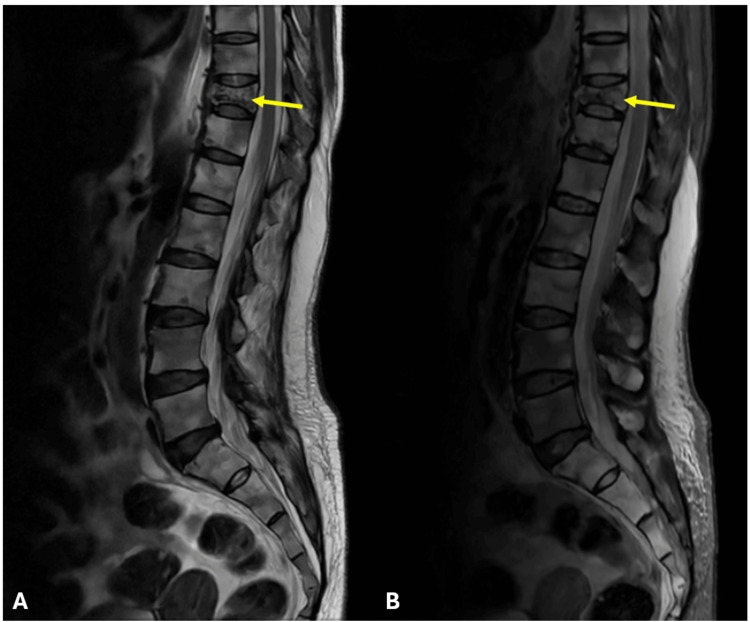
MRI of the spine (sagittal T2 and STIR) demonstrating D10 compression fracture in marginal zone lymphoma Sagittal MRI of the thoracolumbar spine showing (A) T2-weighted image and (B) STIR sequence in a patient with marginal zone lymphoma. The yellow arrow indicates a D10 vertebral compression fracture with marrow infiltration, seen as hypointensity on T2 and hyperintensity on STIR, consistent with lymphoma-associated skeletal involvement. MRI: magnetic resonance imaging; STIR: short tau inversion recovery
